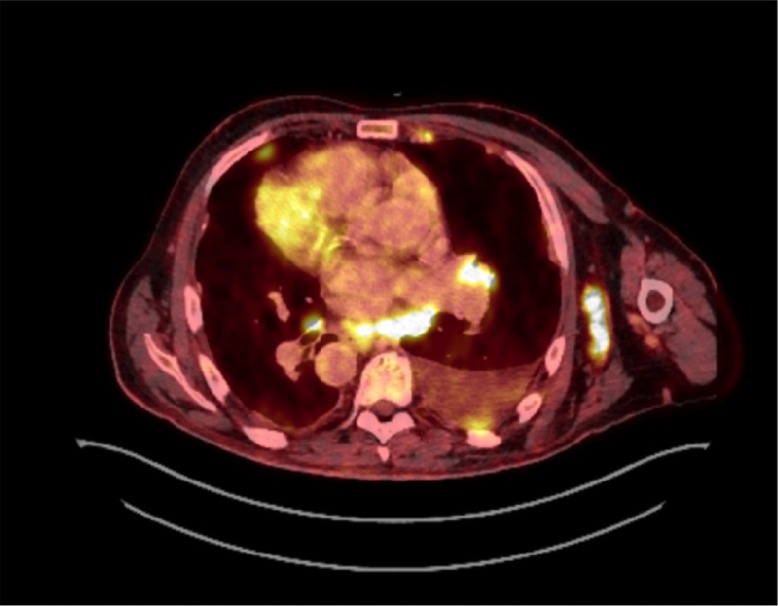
Chest PET-CT scan showing no sign of a primary lung lesion but multiple enlarged lymph nodes, a left-sided compression atelectasis, and ipsilateral pleural effusion.
